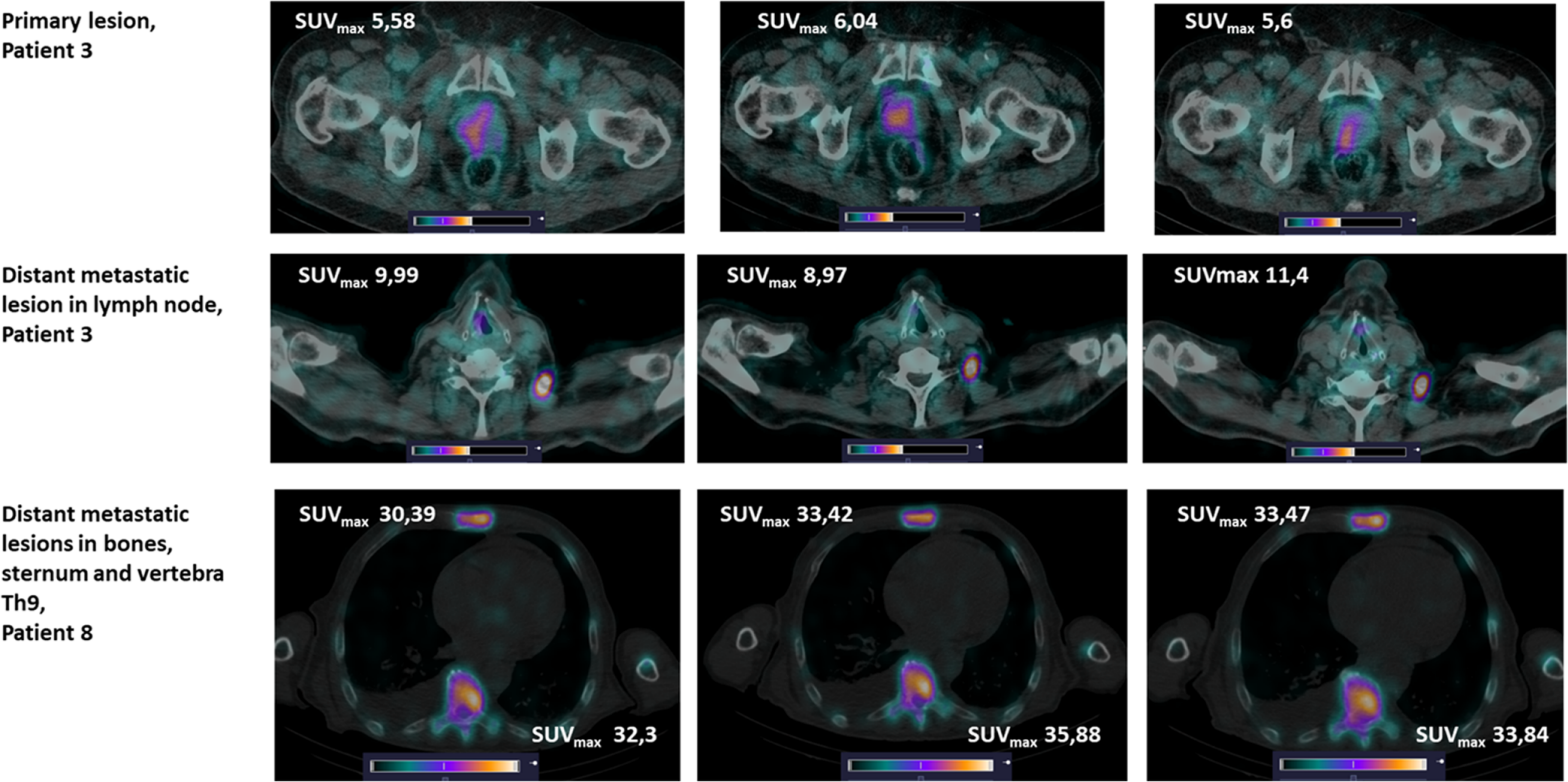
Combined SPECT/CT images of primary lesions
